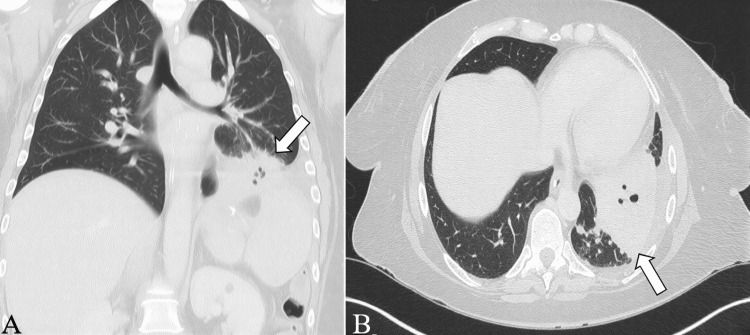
Contrast-enhanced thorax and abdominal CT, arterial phase. (A) Coronal section - Small collection with air foci within noted at the site of the proximal previous sleeve staple line, this collection is continuing into left pleural space collection through the left hemidiaphragm. (B) Axial section - Necrotic left lower lobe
